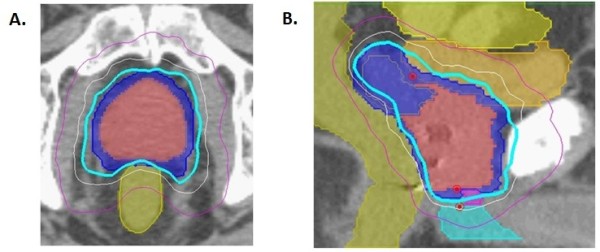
Treatment planning axial (A) and sagittal (B) computed tomography images demonstrating the GTV (red), CTV and PTV expansion (dark blue), bladder (orange), rectum (green), bowel (yellow), membranous urethra (pink) and penile bulb (light blue). Isodose lines shown as follows: Blue 79% (prescription), white 70% and purple 50%.
